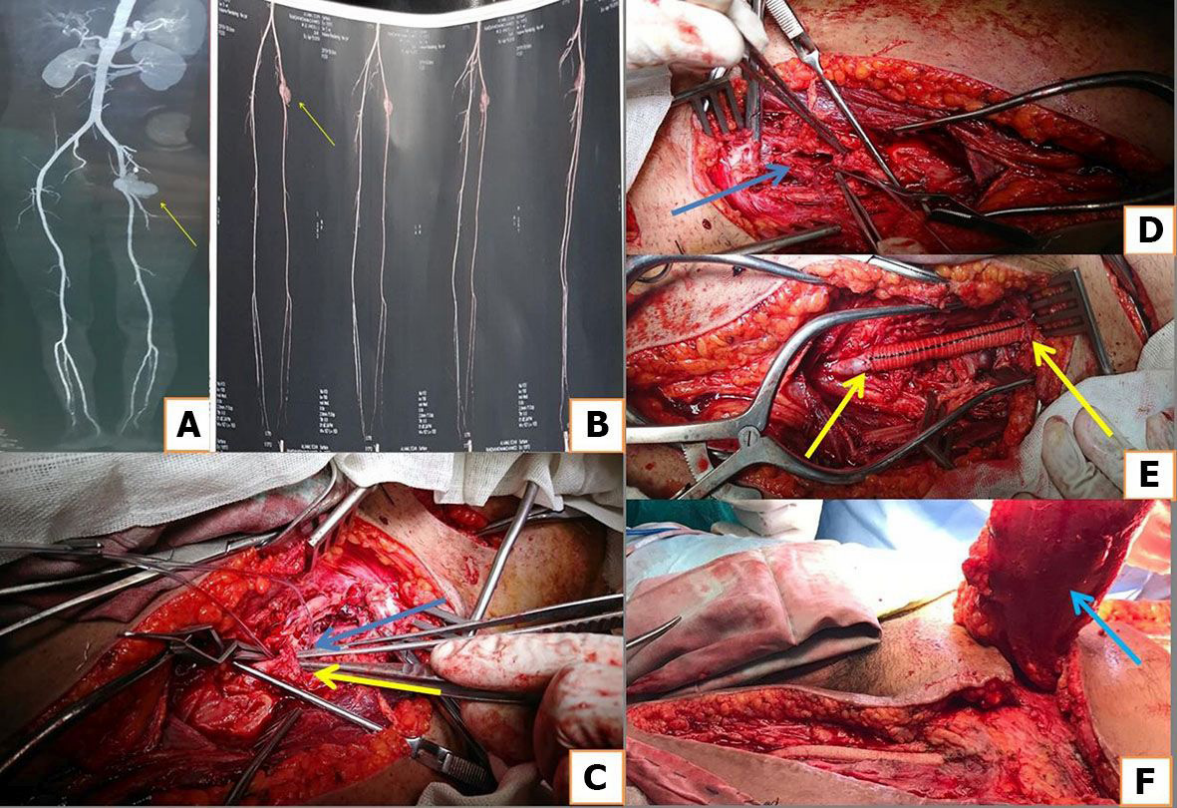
(A, B) Yellow arrow indicates Behçet's pseudoaneurysm of the left common femoral artery, involving the origin of profunda femoris artery, shown by computed tomography angiography (CTA); (C) intraoperative view; blue arrow indicates origin of the profunda femoris artery involved in the pseudoaneurysm; yellow arrow indicates origin of the superficial femoral artery; (D) blue arrow indicates pseudoaneurysm opened after proximal and distal control; (E) yellow arrows indicate proximal and distal anastomoses with 8x60 cm Dacron graft and ligation of the distal profunda femoris artery; (F) blue arrow indicates rectus abdominis flap from contralateral side after 3 secondary hemorrhages and repairs with extension of proximal and distal landings to apparently healthy non diseased artery.
